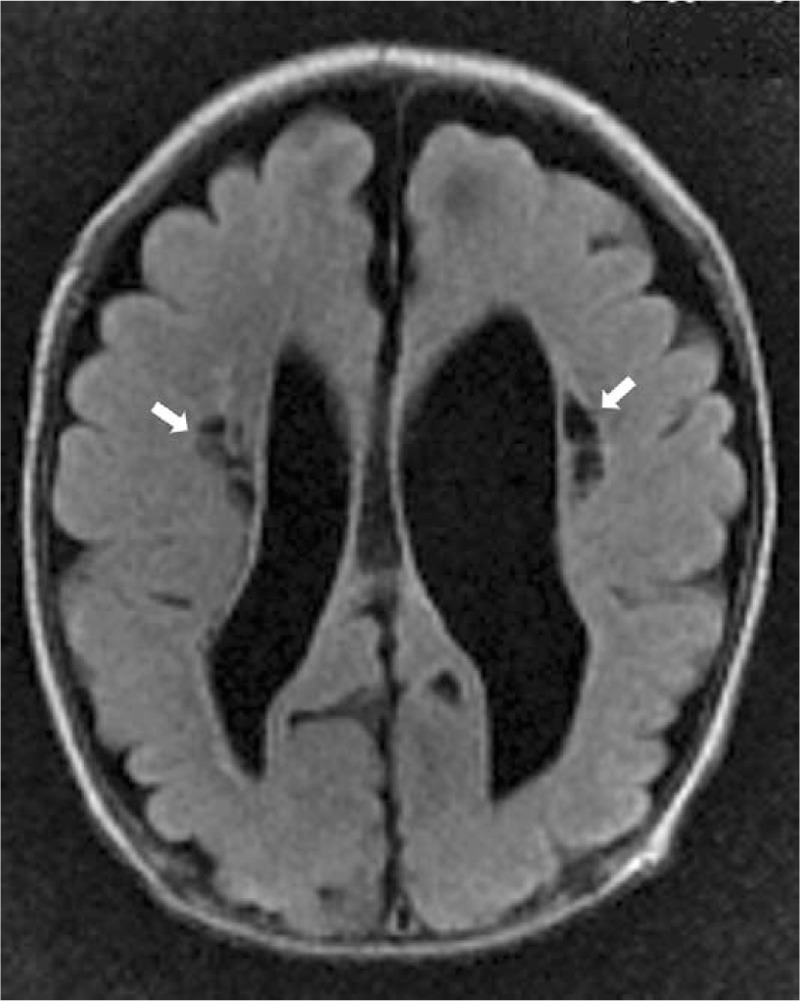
Axial T2-weighted FLAIR magnetic resonance imaging scan at the mid-ventricular level on postnatal day 51 (at 37^+5^ weeks’ postmenstrual age) depicts cystic periventricular leukomalacia, appearing as circumscribed areas of linear and focal low signal intensities (white arrows) within the cerebral white matter abutting the external angles of the lateral ventricles, and asymmetric enlargement of the lateral ventricles (more prominent on the left). FLAIR = fluid-attenuated inversion recovery.
